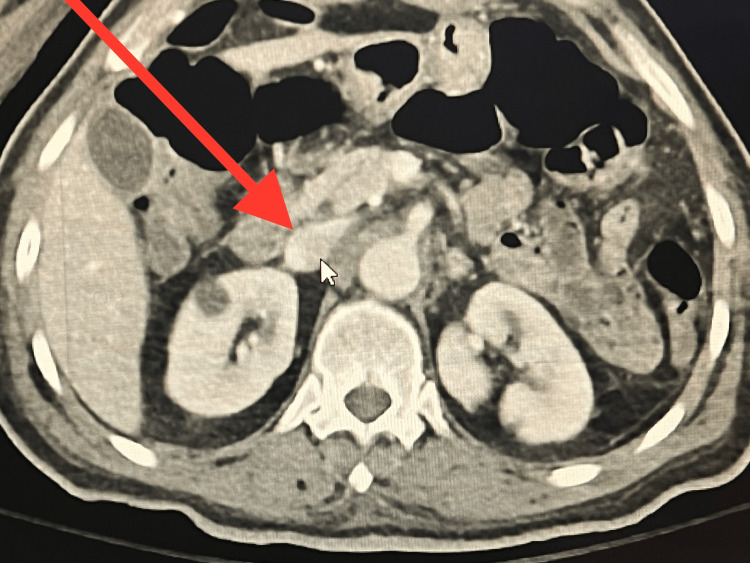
Abdominal CT scan showing distention of the right renal vein and significant distention of the suprarenal vena cava.
